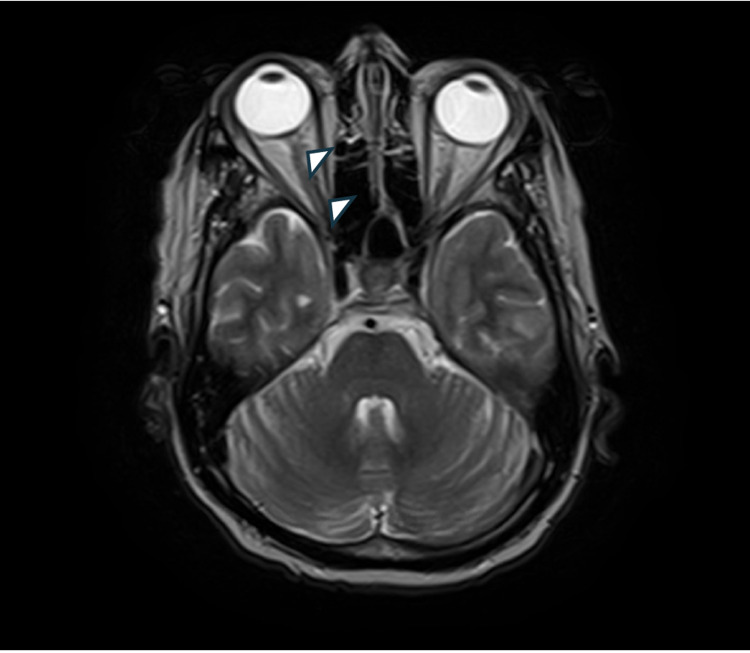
Arrowhead: axial T2-weighted gadolinium-enhanced MRI demonstrates hyperintense signal with heterogeneous enhancement along the right optic nerve sheath
